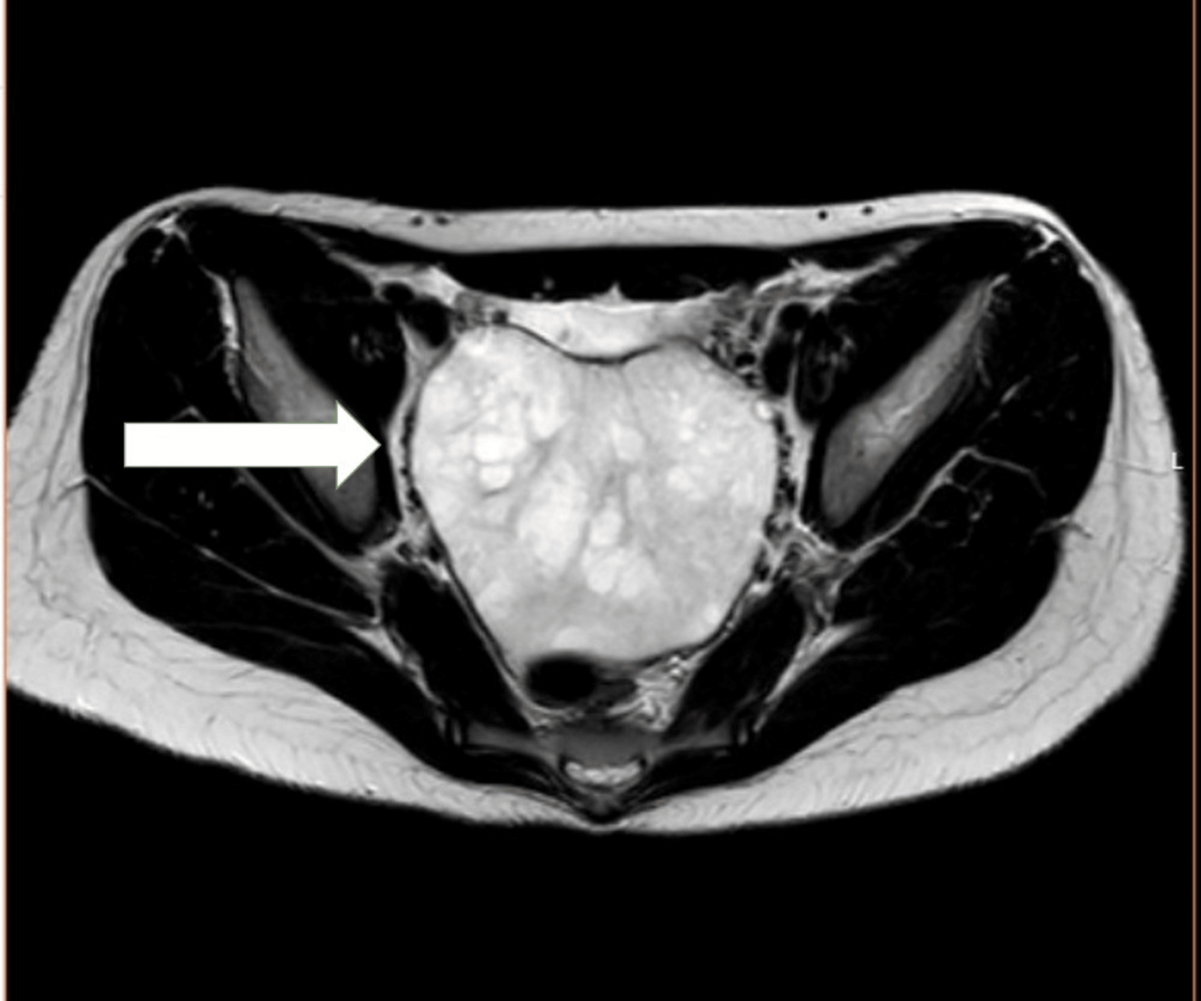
Pelvic MRI showing adnexal mass in axial (transverse) section
